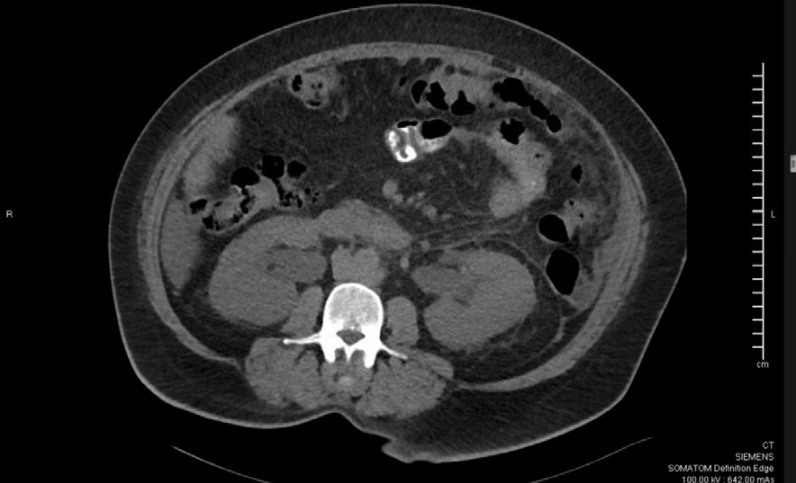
Computed tomography scan showing bilateral hydronephrosis
